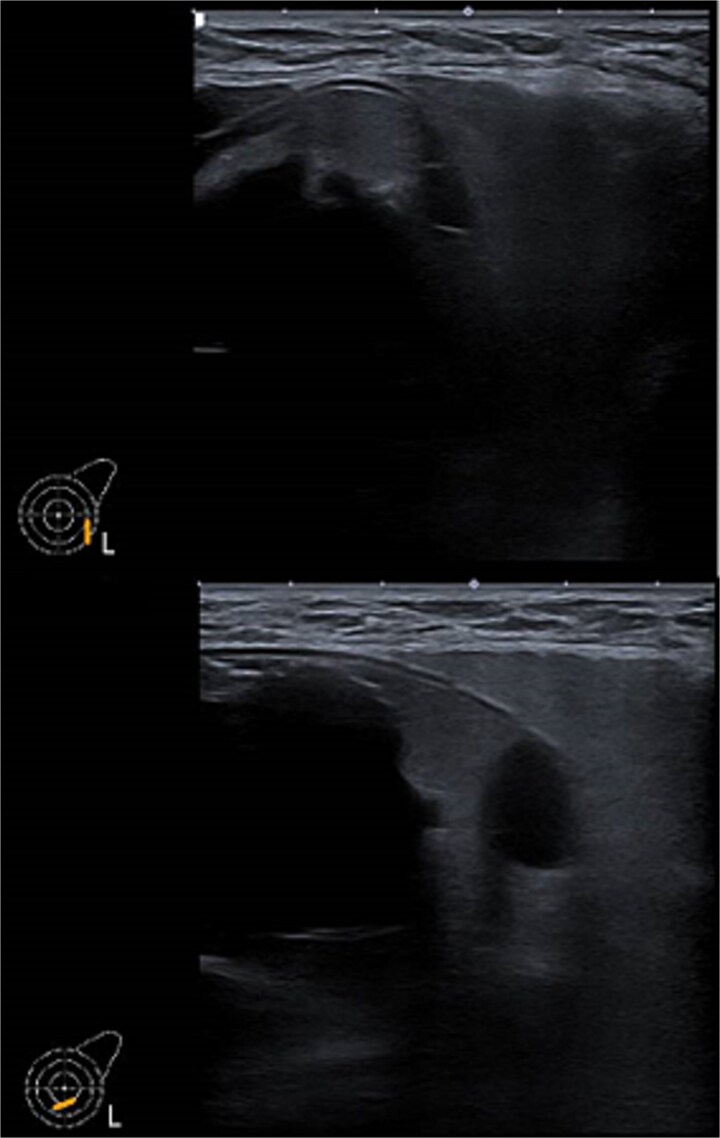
Ultrasound of the left breast showed rupture of the left breast implant with intracapsular and extracapsular leakage, surrounded by echogenic peri-implant fluid.
